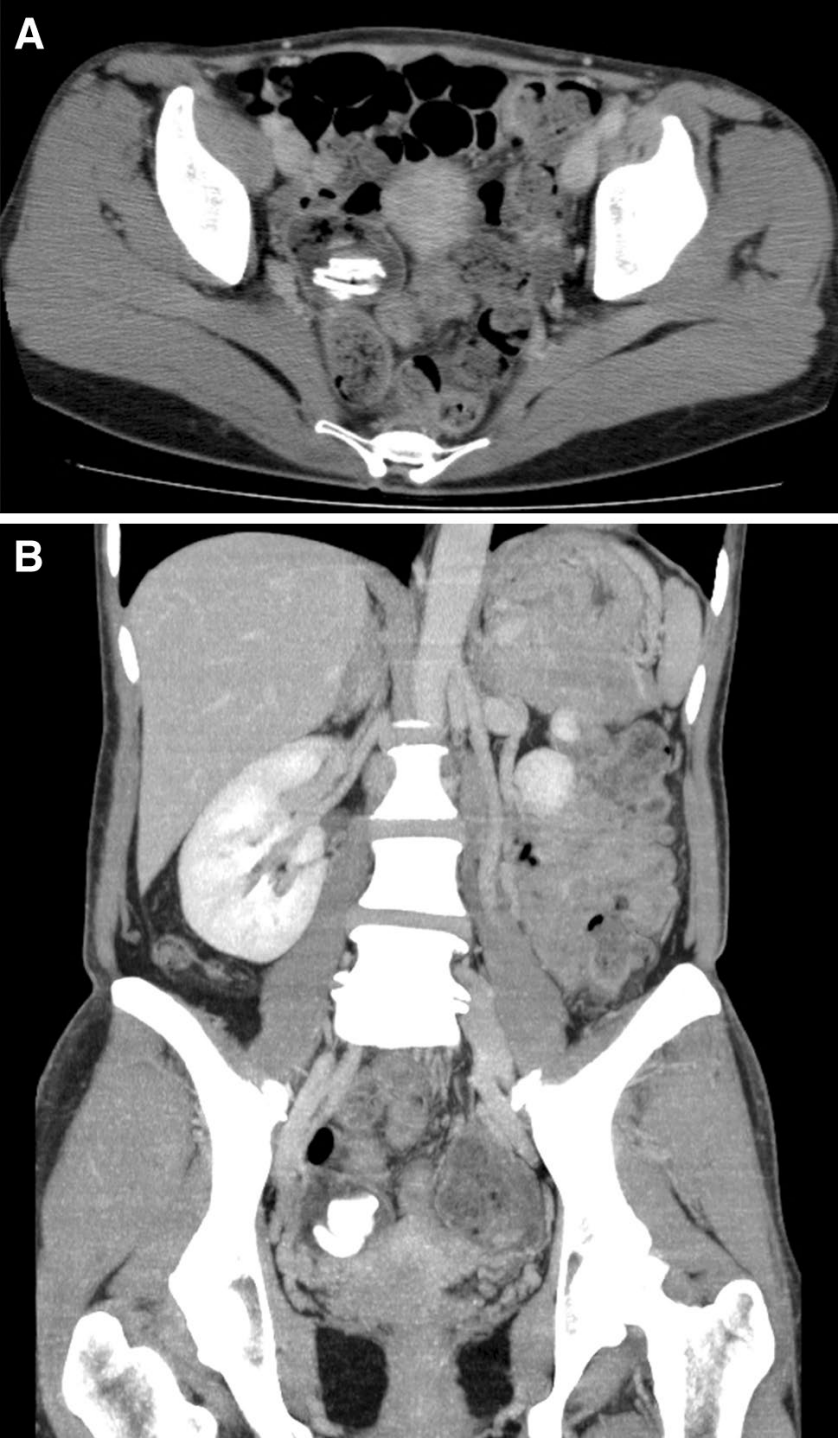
Abdominal enhanced CT showing a 40-mm mass containing air and linear high-density areas. The capsule of the mass had a slight enhancement effect. a Axial image, b coronal image
